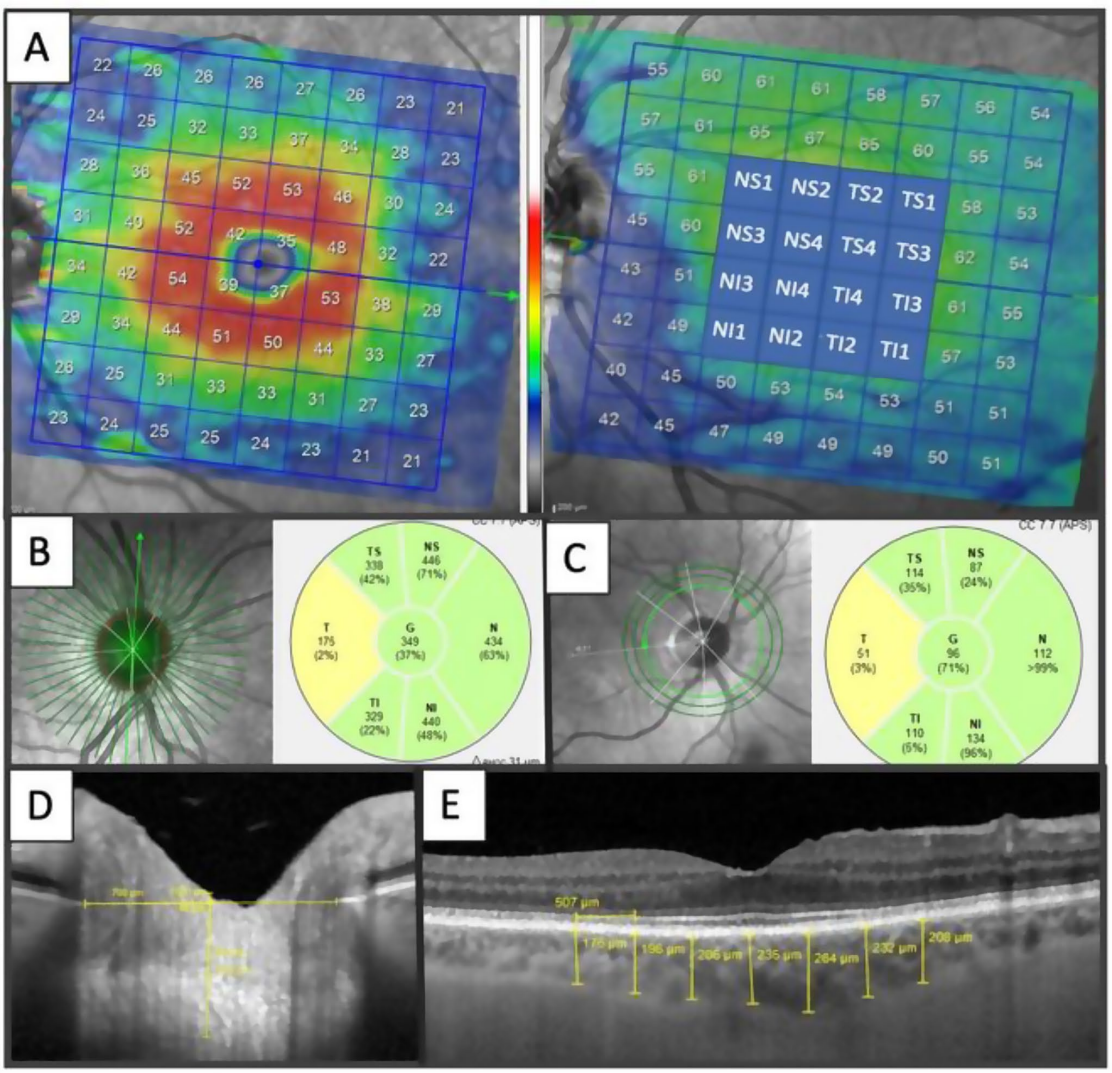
Optical coherence tomography parameters. A: Ganglion Cell Layer (GCL). B: Bruch’s membrane opening–minimum rim width (BMO-MRW). C: Retinal nerve fiber layer (RNFL), D: Lamina cribrosa (LC), E: Choroidal thickness (CT)
